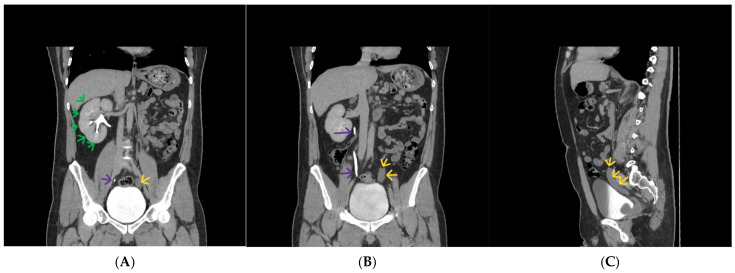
(A–C) Coronal and sagittal reformatted CT images, delayed phase, showing hypertrophied right kidney (green arrows) and absent left kidney. Left ureter with proximal blind-ending (yellow arrows). Normal right ureter (purple arrows). Normal excretion of contrast by right kidney with accumulation of contrast in urinary bladder.
